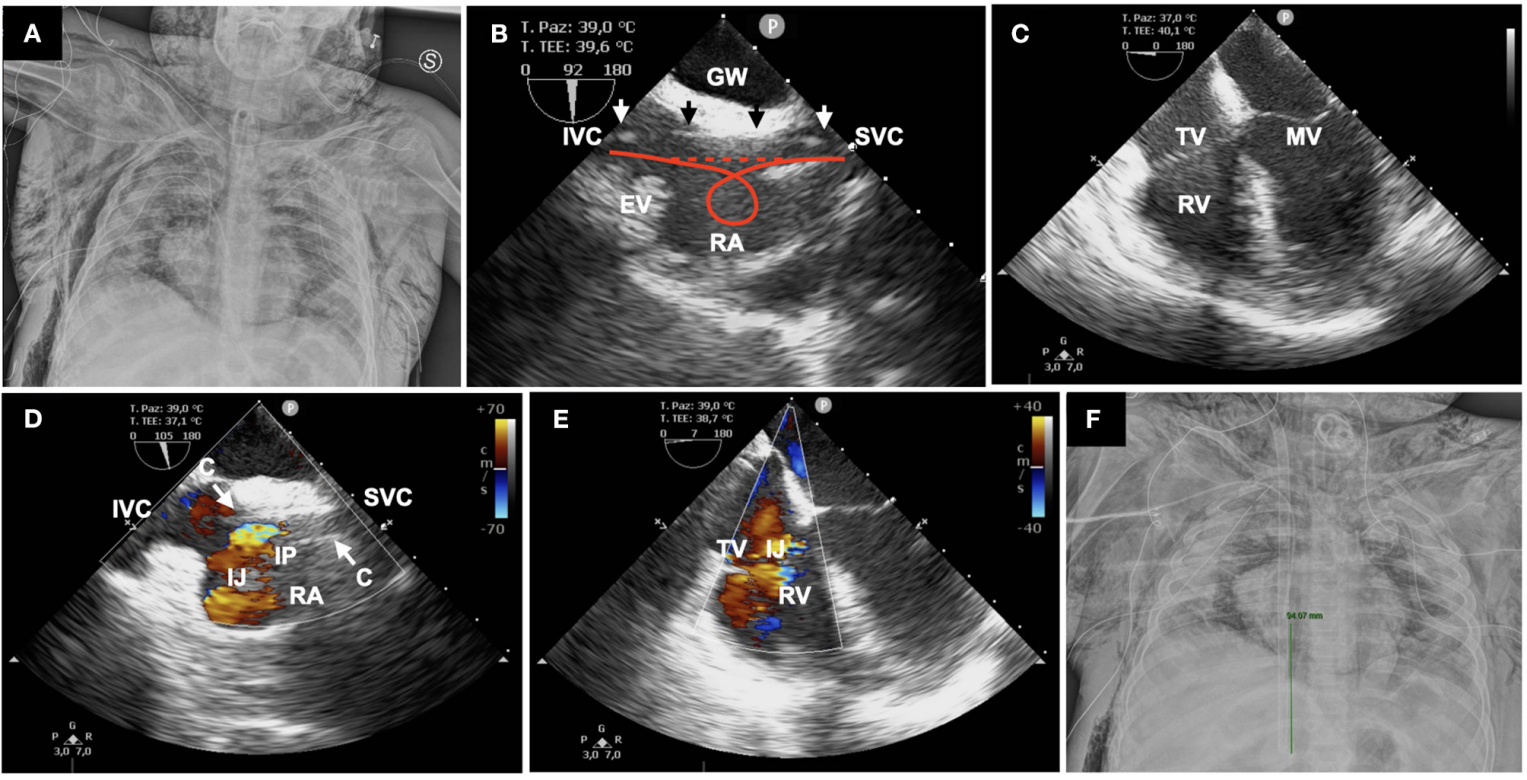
(A) Massive air leak syndrome with pneumomediastinum, bilateral pneumothorax, and subcutaneous emphysema. (B) GW (arrows) displayed straight from the SVC to IVC; the red lines show how a discontinuous hyperechoic image can help in ruling out looping; right atrium (RA), Eustachian valve (EV). (C) Absence of looping or GW malposition in the right ventricle (RV) is demonstrated in four-chamber MEV; tricuspid valve (TV), mitral valve (MV). (D) Mid-esophageal bicaval view showing the correct position of the cannula (C) with the infusion port (IP) in the middle of the right atrium; infusion jet (IJ). (E) Four-chamber MEV confirming the direction of the IJ toward the tricuspid valve. (F) Chest X-ray demonstrating the correct position of the cannula; the green line measures the tip-to-IP distance (9.4 cm).
